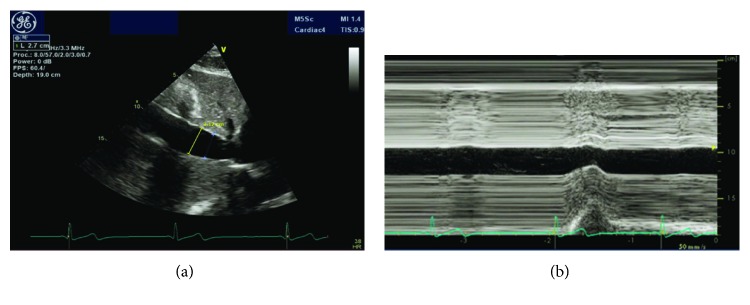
(a) IVC on transthoracic echocardiogram. Dilated IVC measuring 3.12 cm. (b) IVC on M-mode. M-mode with inspiration with maximum diameter measuring 3.12 cm and minimum diameter of 2.5 cm.
